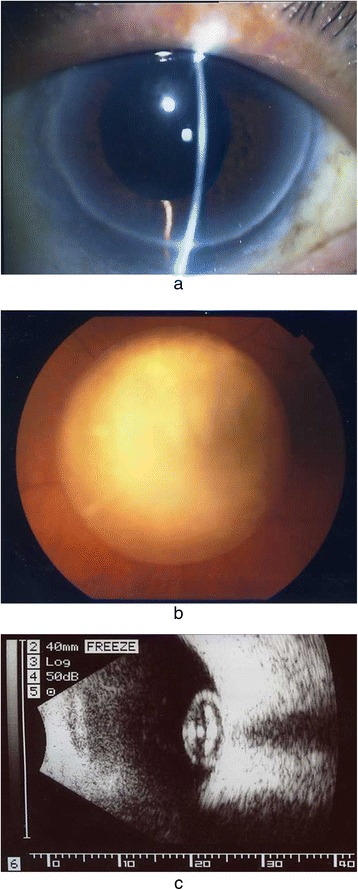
a Preoperative anterior segment of the patient’s left eye. Slit-lamp examination revealed absence of the crystalline lens and deep anterior chamber. b Preoperative fundus image of the left eye. The luxated lens in the vitreous cavity can be seen stuck to the optic disc. The patient’s visual acuity in that eye was 20/400. c B-mode ultrasonography findings. No movement of the luxated lens was observed in relation to changes in the patient’s head position or eye movement
